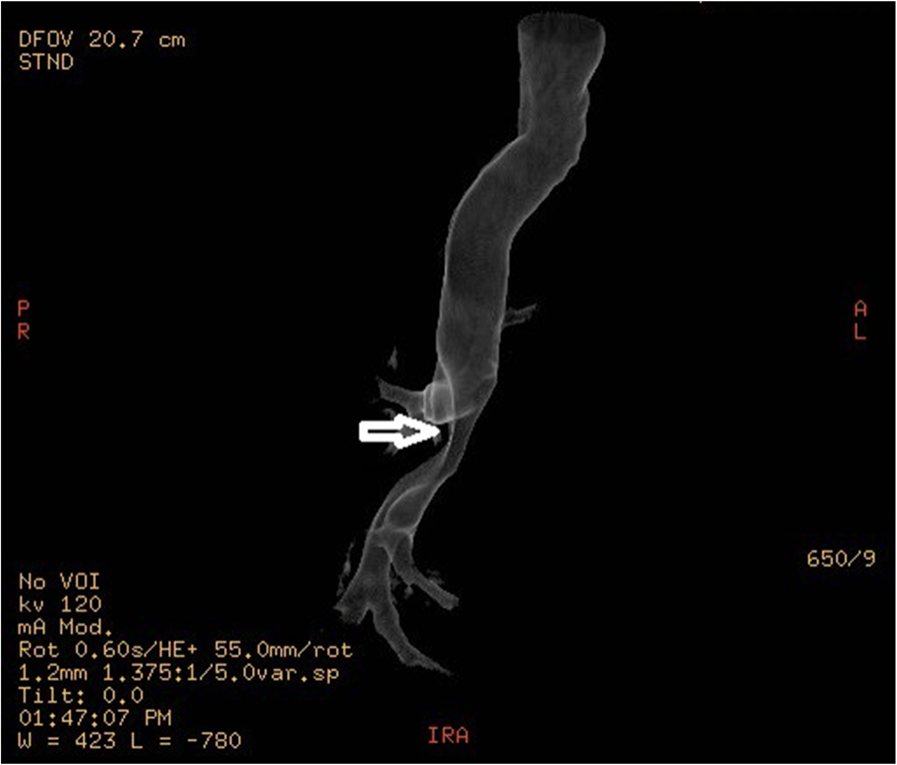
Spiral computed tomography showed that the tumor extended just across the carina (arrow).
